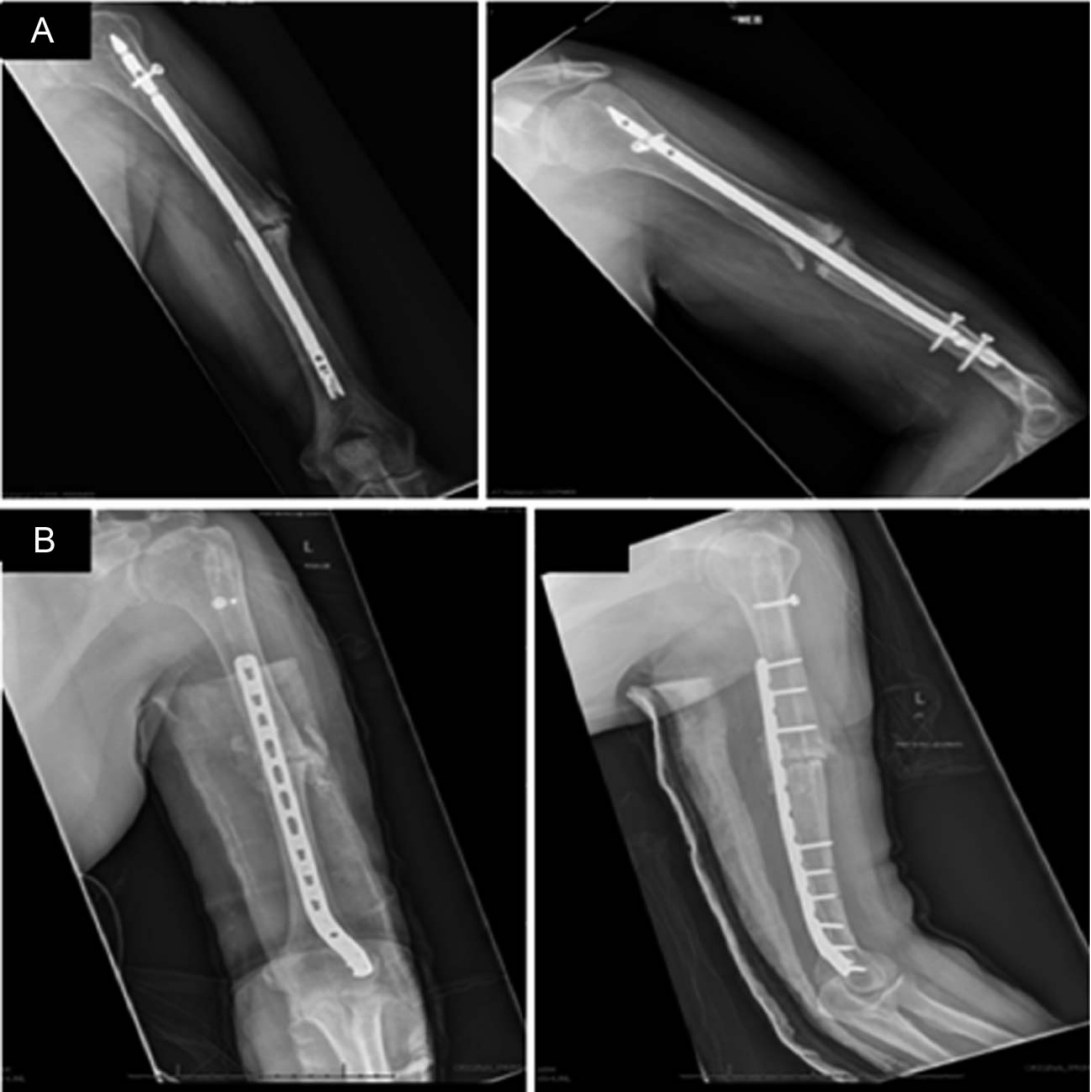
Preoperative radiographs of the atrophic humeral nonunion (A) and postoperative radiographs after compression plating, debridement with acute shortening, and iliac crest bone graft (B).
